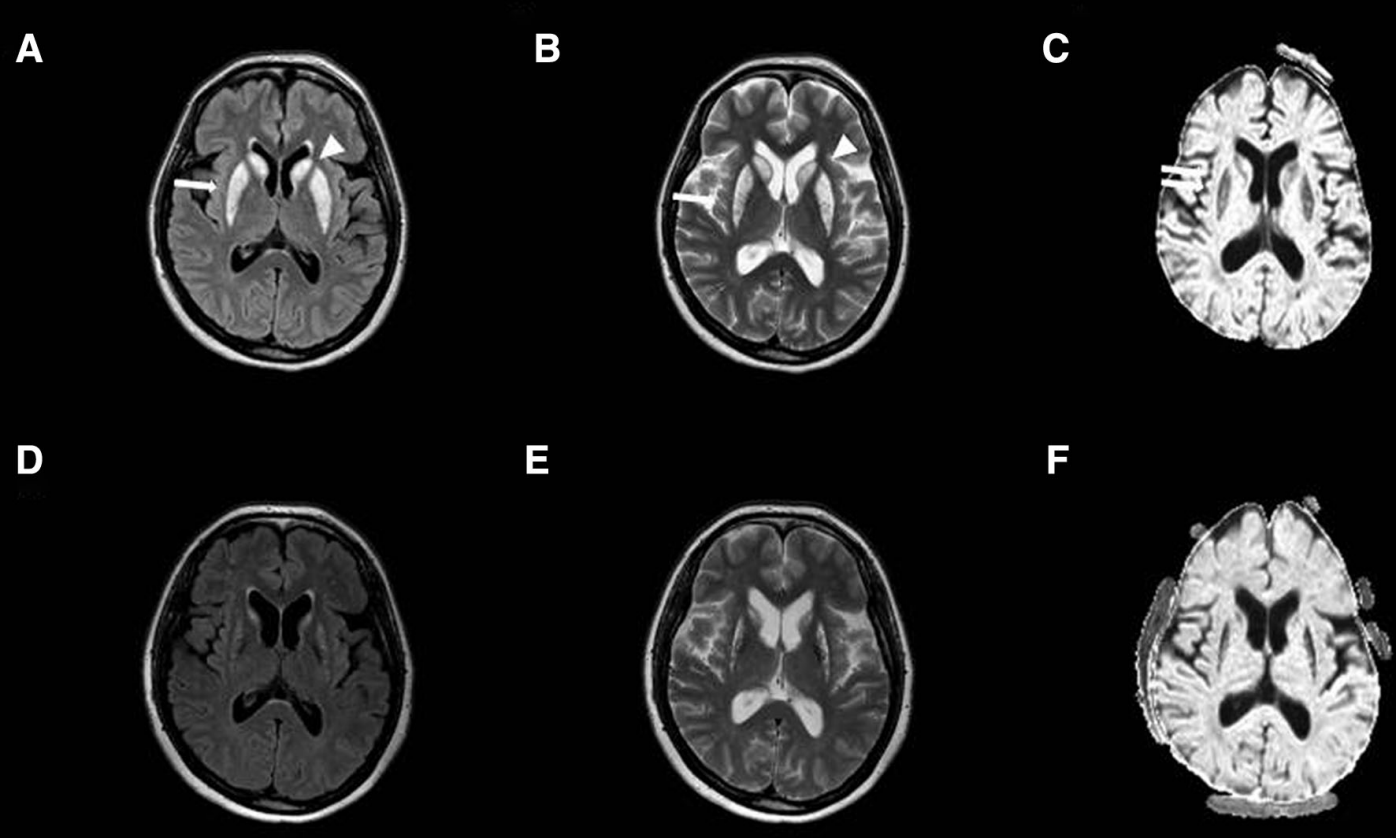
Axial brain imaging in the acute phase, showing increased signal intensity in T2 (a) and FLAIR (b) imaging of the nuclei caudatus (*arrow head*) and putamen (*arrow*) with sparing of the globus pallidus. DWI/ADC imaging in c with diffuse restriction in putamen matching ischemic infarction (*double arrows*). Axial brain imaging after 2 years follow-up, showing gliosis in T2/FLAIR and DWI/ADC (d–f) of the nuclei caudatus and putamen
